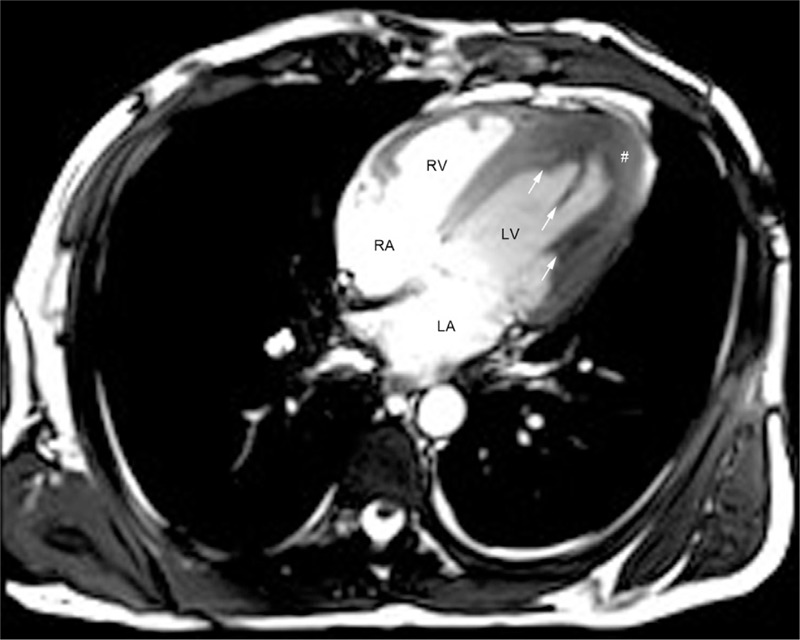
Single image of cine-MRI with True FISP sequence in the LV 4-chamber long axis plane of the second patient (white arrows indicate the borders of the thrombus; # delineates the thickened endocardium). LV = left ventricular, MRI = magnetic resonance imaging, True FISP = true fast imaging with steady-state precession.
